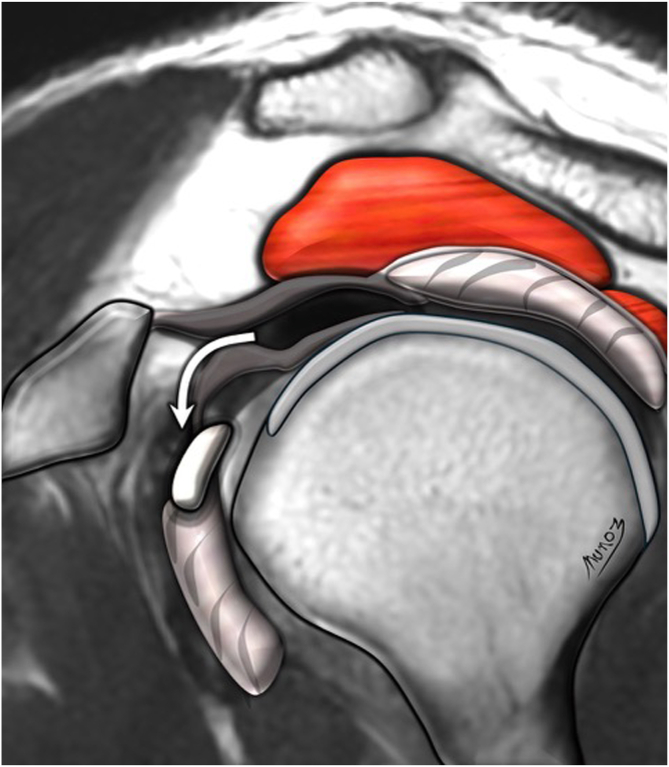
Displacement sign (white curved arrow) is shown in a sagittal view of a T1-weighted right shoulder MRI. (MRI, magnetic resonance imaging.)
